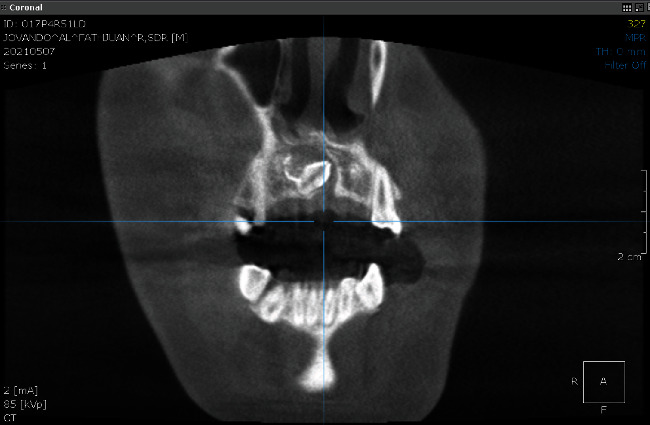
CBCT radiographic view. The mesiodens impacted tooth was seen between teeth 11 and 21 in an inverted position. Mesiodens tooth distance with the base of the nasal cavity: 3.93 mm. A mixed radiolucent-radiopaque image is seen around the crown of the mesiodens with a clear border with radiopaque edges and an oval shape. The lesion appears to cause a discontinuity of the palatal cortical bone, and the lesion is in contact with the floor of the nasal cavity.
